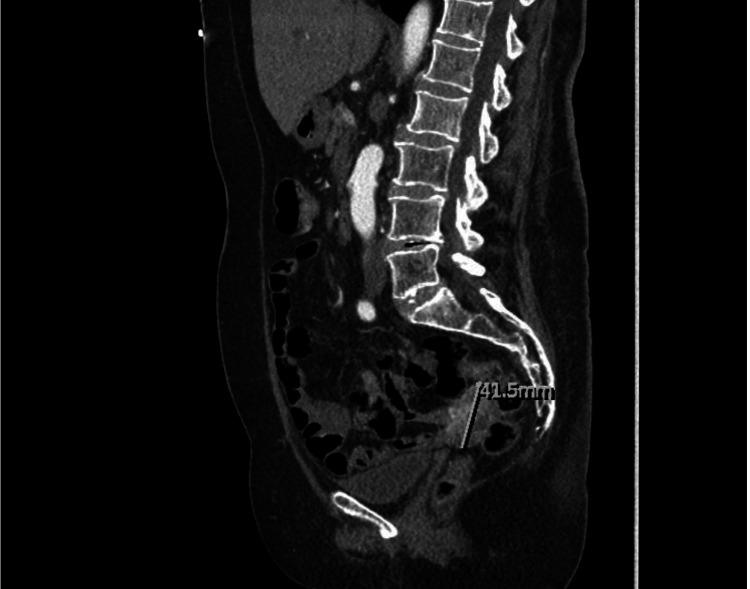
CT angiogram showing a heterogeneously arterial phase hyper-enhancing exophytic circumscribed lesion involving the ileum, measuring 4.7 x 3 x 4.1 cm
